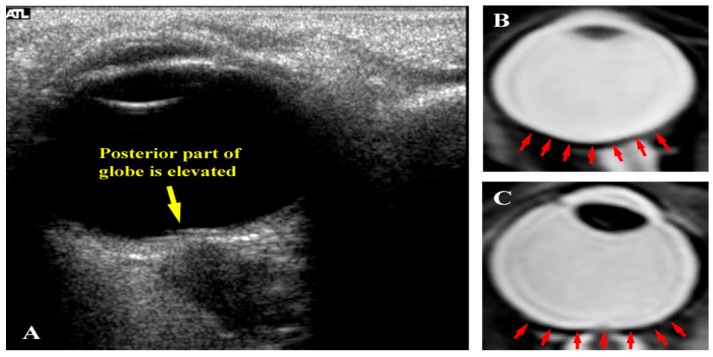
(a) Inflight ultrasound of a globe showing flattening of the posterior globe; (b) Magnetic resonance image (MRI) of a normal globe before long-duration spaceflight; and (c) MRI of a globe after a long-duration flight exhibiting posterior flattening. Source: NASA Longitudinal Study of Astronaut Health.
